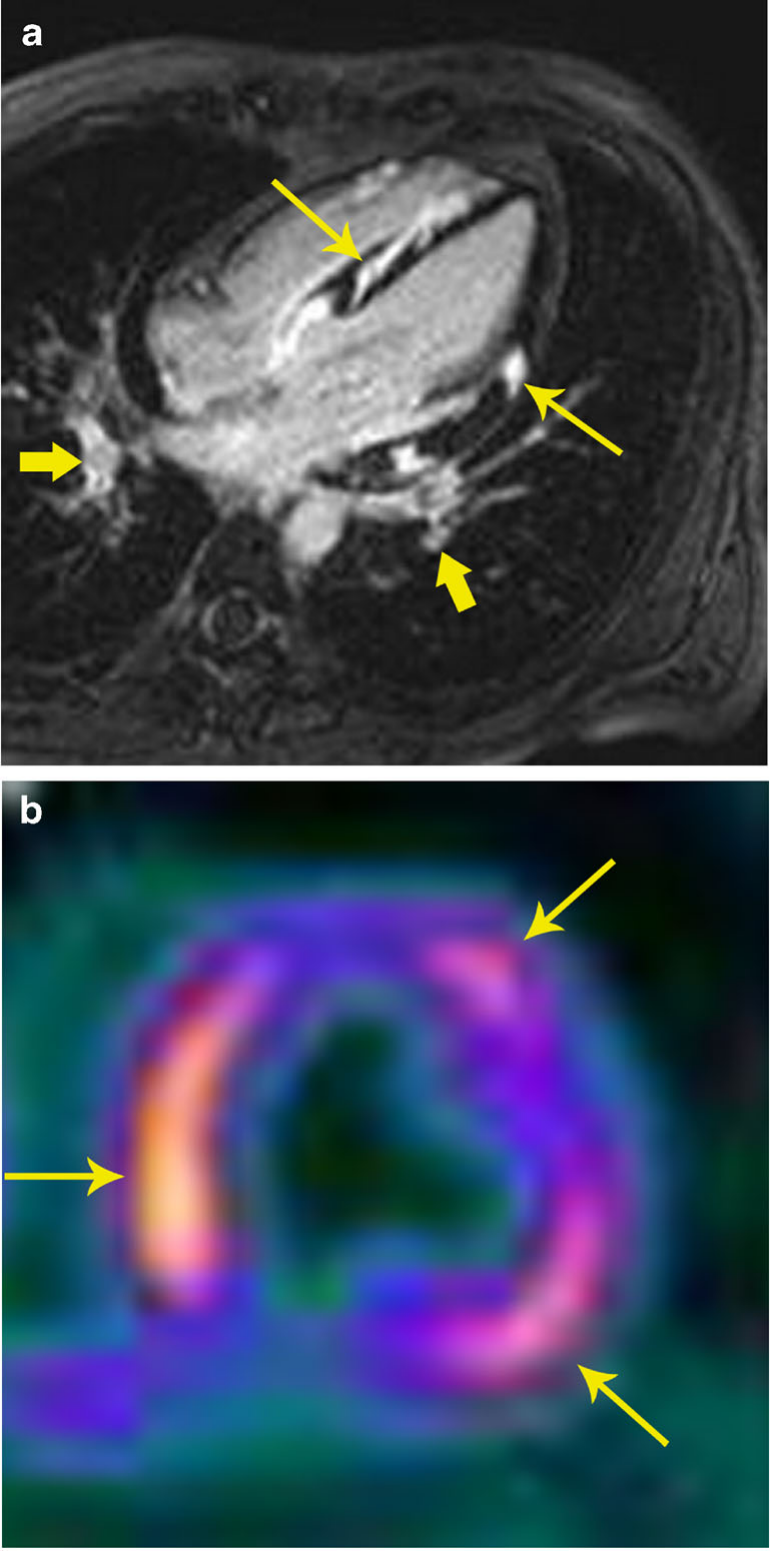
Sarcoidosis. a 32-year-old male admitted after a syncopal episode while shoveling snow. He was diagnosed with pulmonary sarcoidosis by transbronchial biopsy. Four-chamber delayed enhancement MRI shows patchy, diffuse areas of subepicardial as well as transmural areas of delayed post-contrast enhancement involving both ventricles (thin arrows). Bilateral hilar adenopathy was also seen (thick arrows). b FDG- PET after a fatty diet shows patchy areas of high uptake in the myocardium indicating areas of active inflammation
